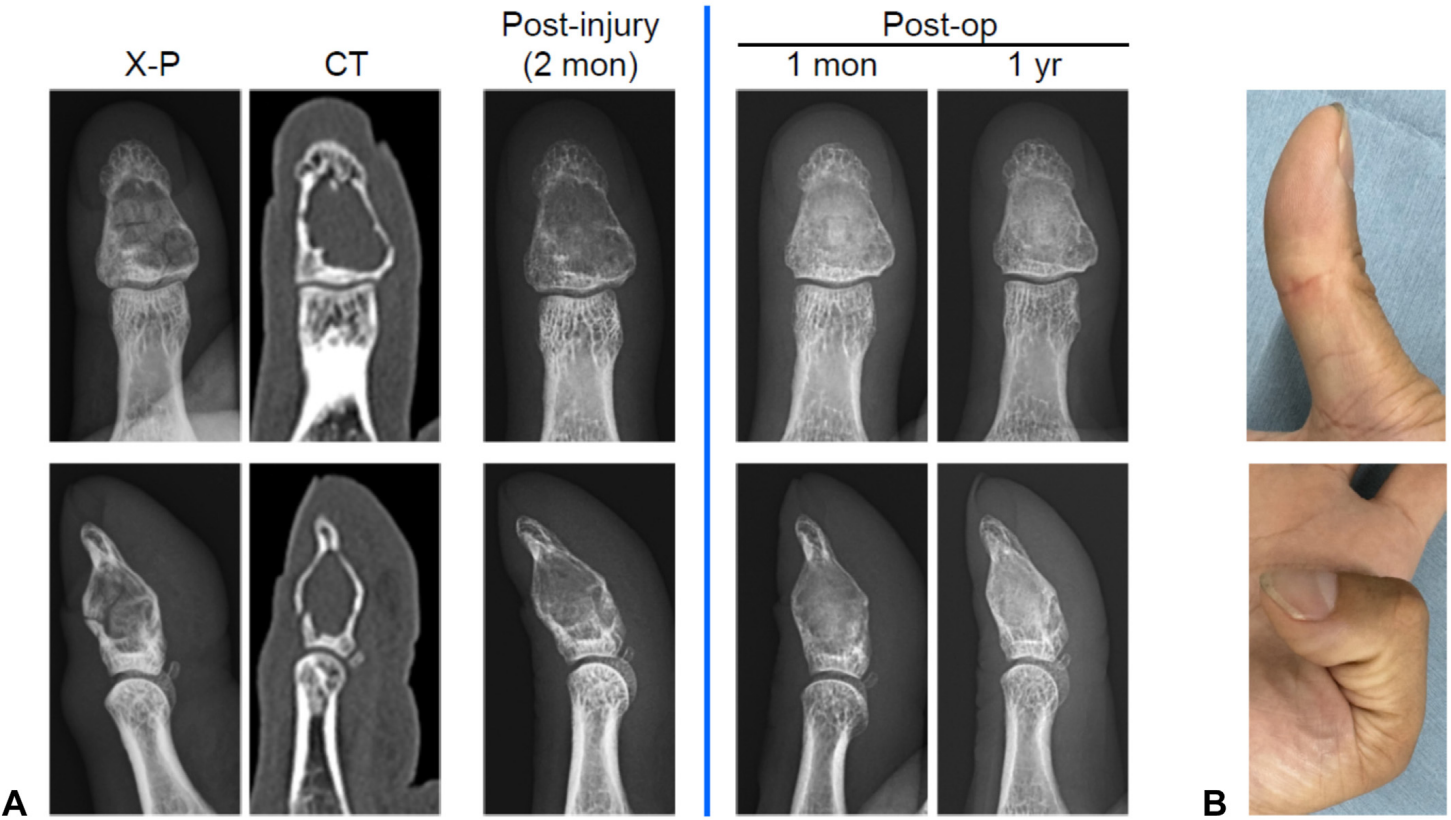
Pre- and postoperative findings for case 2. A Left of the blue line: initial radiographs, computed tomography, and postimmobilization radiographs at 2 months, showing frontal (upper row) and lateral (lower row) views. Right of the blue line: postoperative radiographs at 1 month and 1 year, showing frontal (upper row), and lateral (lower row) views. B Thumb range of motion at 1 year after surgery: extension (upper) and flexion (lower).
